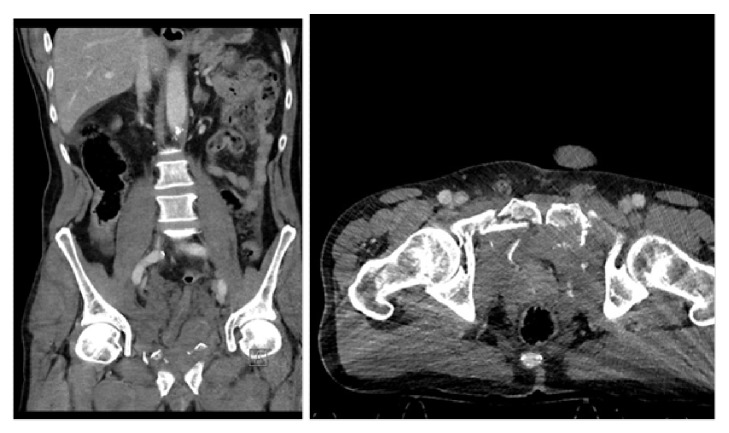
Contrast-enhanced CT: pelvic fracture and pelvic hematoma. The bladder cannot be assessed.
